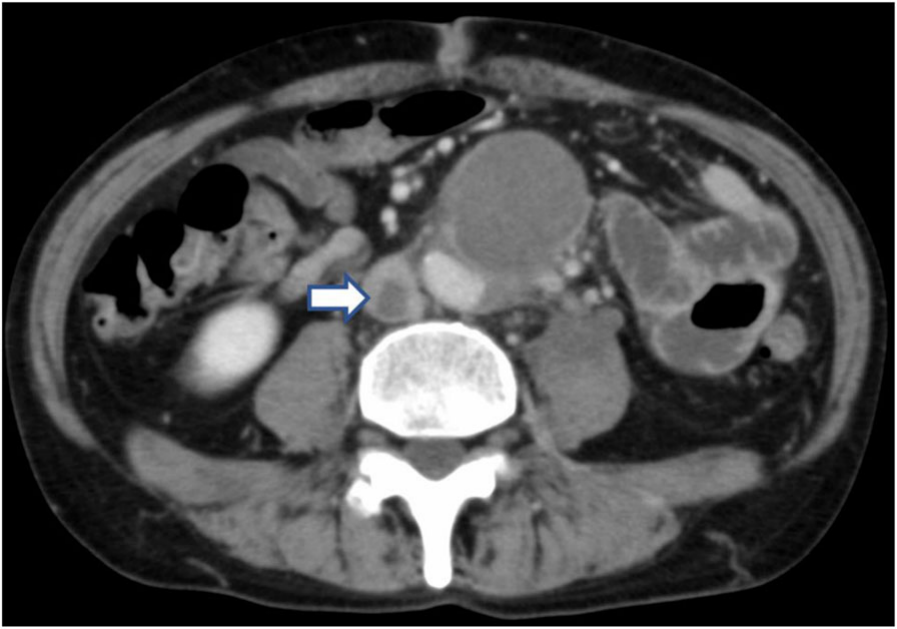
Enhanced computed tomography at initial examination showing the 10 mm thrombus in the inferior vena cava (arrow)
